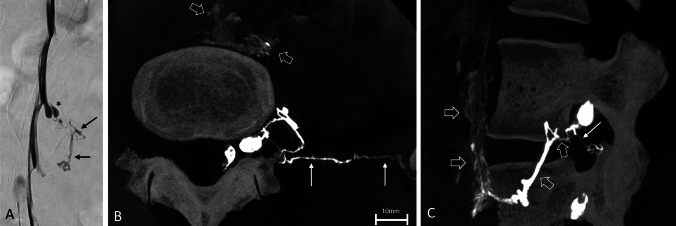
CSF-lymphatic fistula demonstrated by 2D and 3D myelography techniques. Digital subtraction myelography (DSM) in left lateral decubitus position shows an egress of contrast medium, exiting from a small nerve root diverticulum (asterisks in A) at the level L4/5 on the left into irregular shaped para-spinal vessels (black arrows in A). Ultrahigh-resolution cone-beam CT (UHR-CBCT) myelography depicts a CSF-lymphatic fistula (CLF) with two main drainage pathways: a ventral drainage along paravertebral and retroperitoneal lymphatic vessels (open white arrows in B and C) and a lateral drainage into the left abdominal trunk (solid white arrows in B and C)
